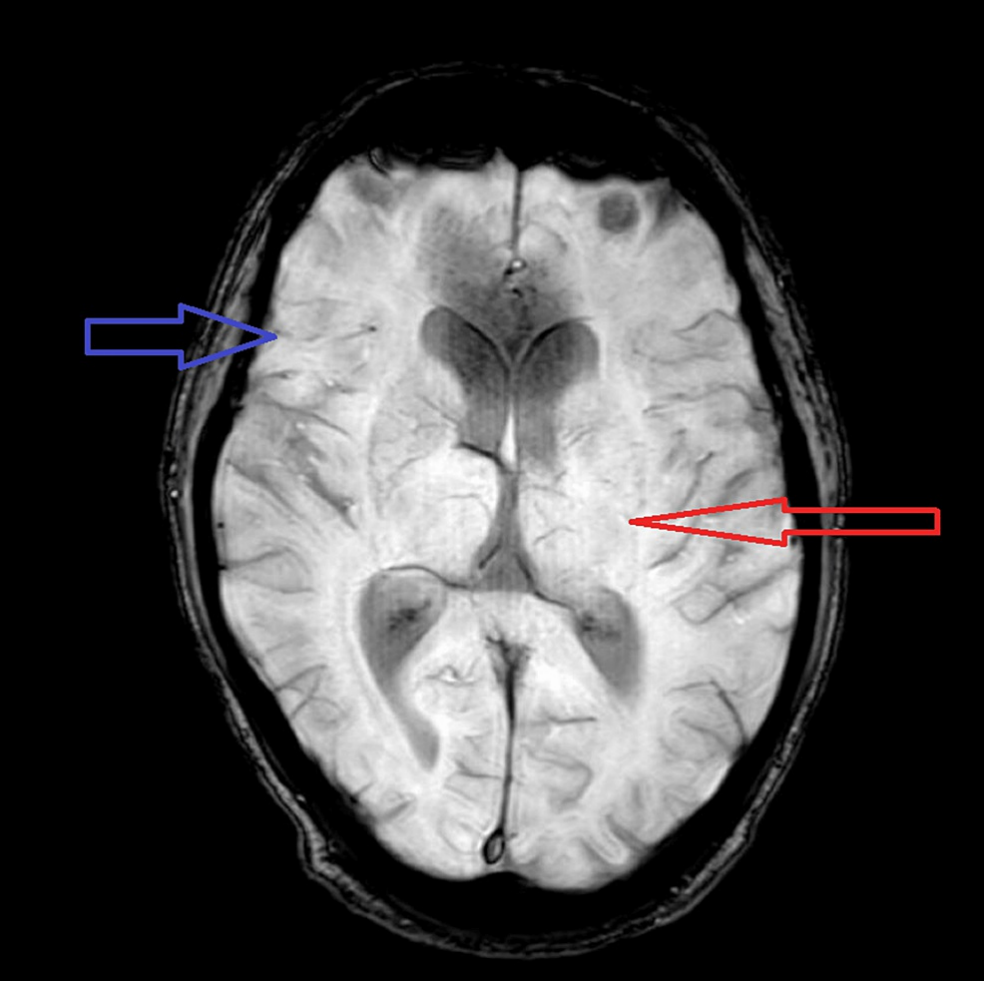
MRI Scan Showing Age-Related Atrophic Changes and Periventricular Hyperintensity MRI, magnetic resonance imaging
